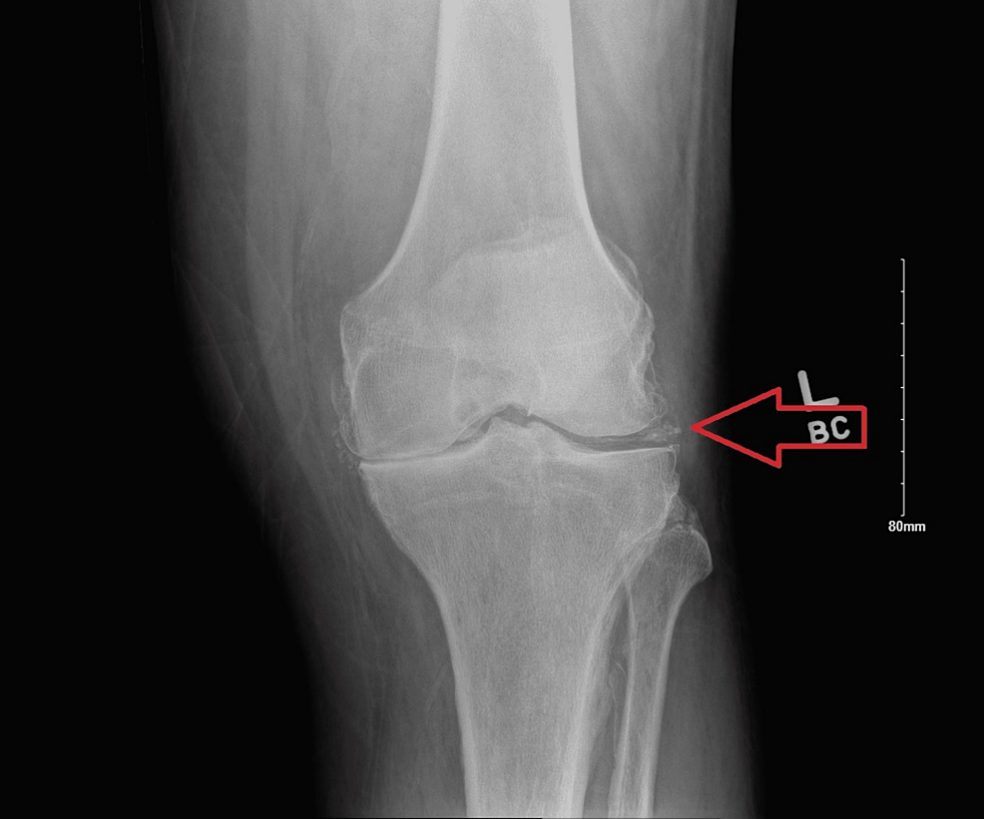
Left knee X-ray anteroposterior incidence showing signs of osteoarthritis (joint space narrowing and osteophyte formation) and chondrocalcinosis (red arrow).
